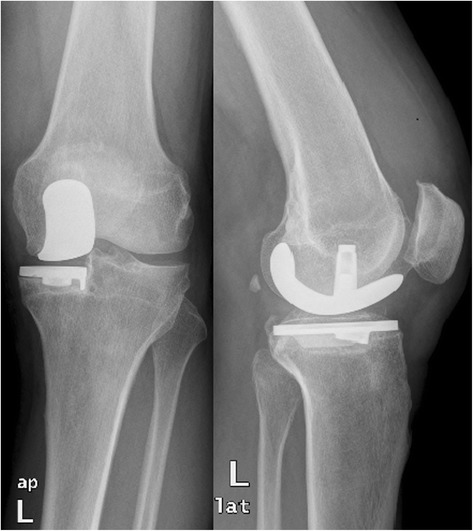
X-rays (AP and lateral view) of loosened implants of unicompartmental knee arthroplasty
